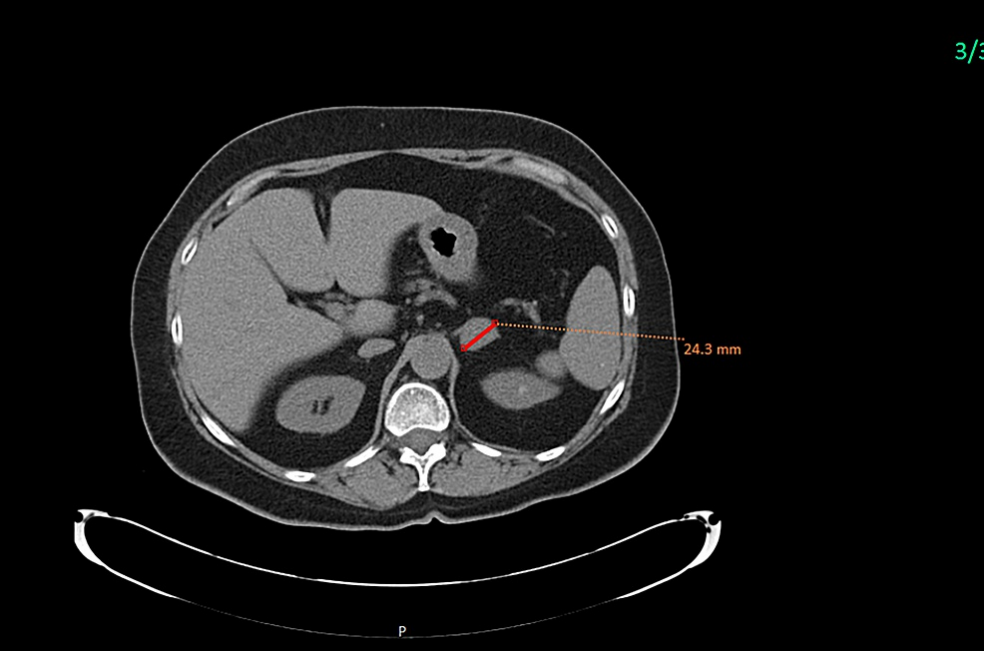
Initial CT image showing the apparent benign left adrenal incidentaloma, which is homogenous, rounded, and has a low density measuring 24.1 mm (maximum diameter). CT: computed tomography
